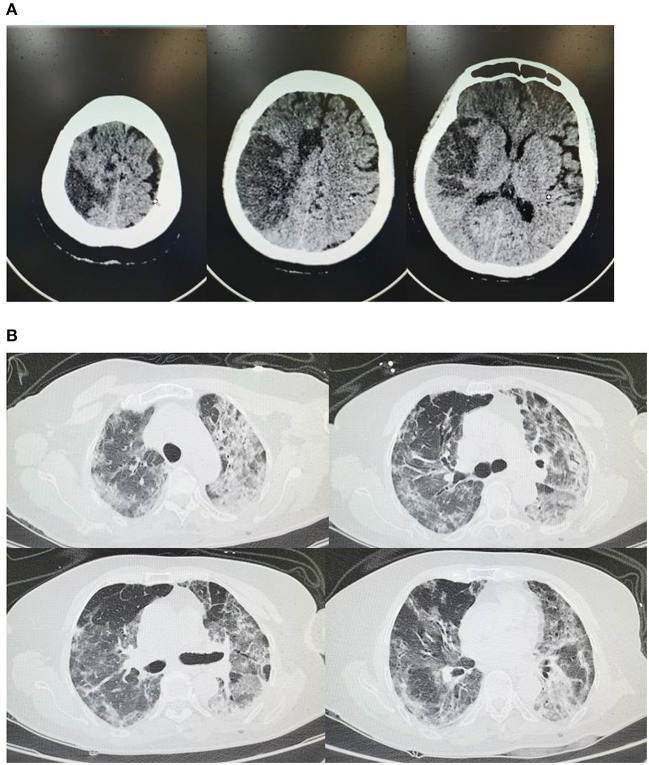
(A) On February 20th head CT shows bilateral cerebral infarcts involving the middle and anterior cerebral artery distribution on the right and anterior cerebral artery on the left; (B) On February 20th chest CT shows bilateral interstitial ground glass-like shadows and multiple consolidations.
